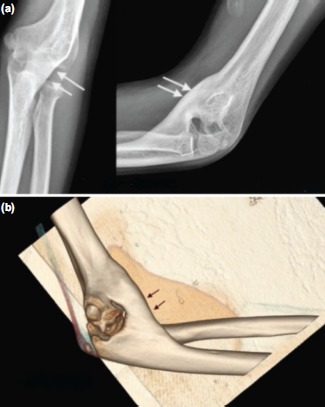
(a) Antero-posterior and Lateral radiographs showing HO Hastings IIIA bridging the humero-ulnar joint anteromedially (arrows), (b) 3D CT of case shown in Figure 1a.
